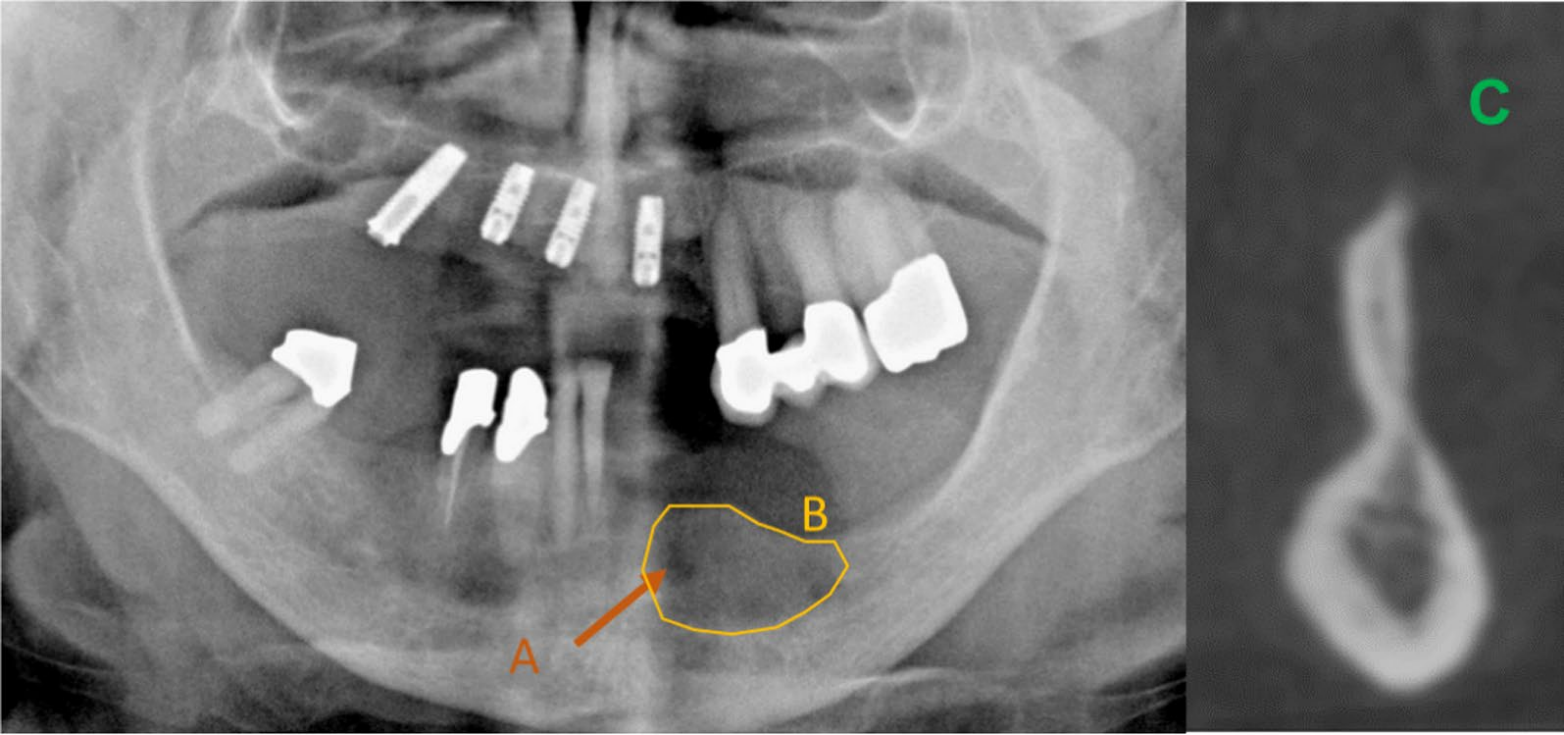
Panoramic radiography image showing A a distinct hypodense lesion and B a translucent area. The depiction of the same region in CBCT (C)
